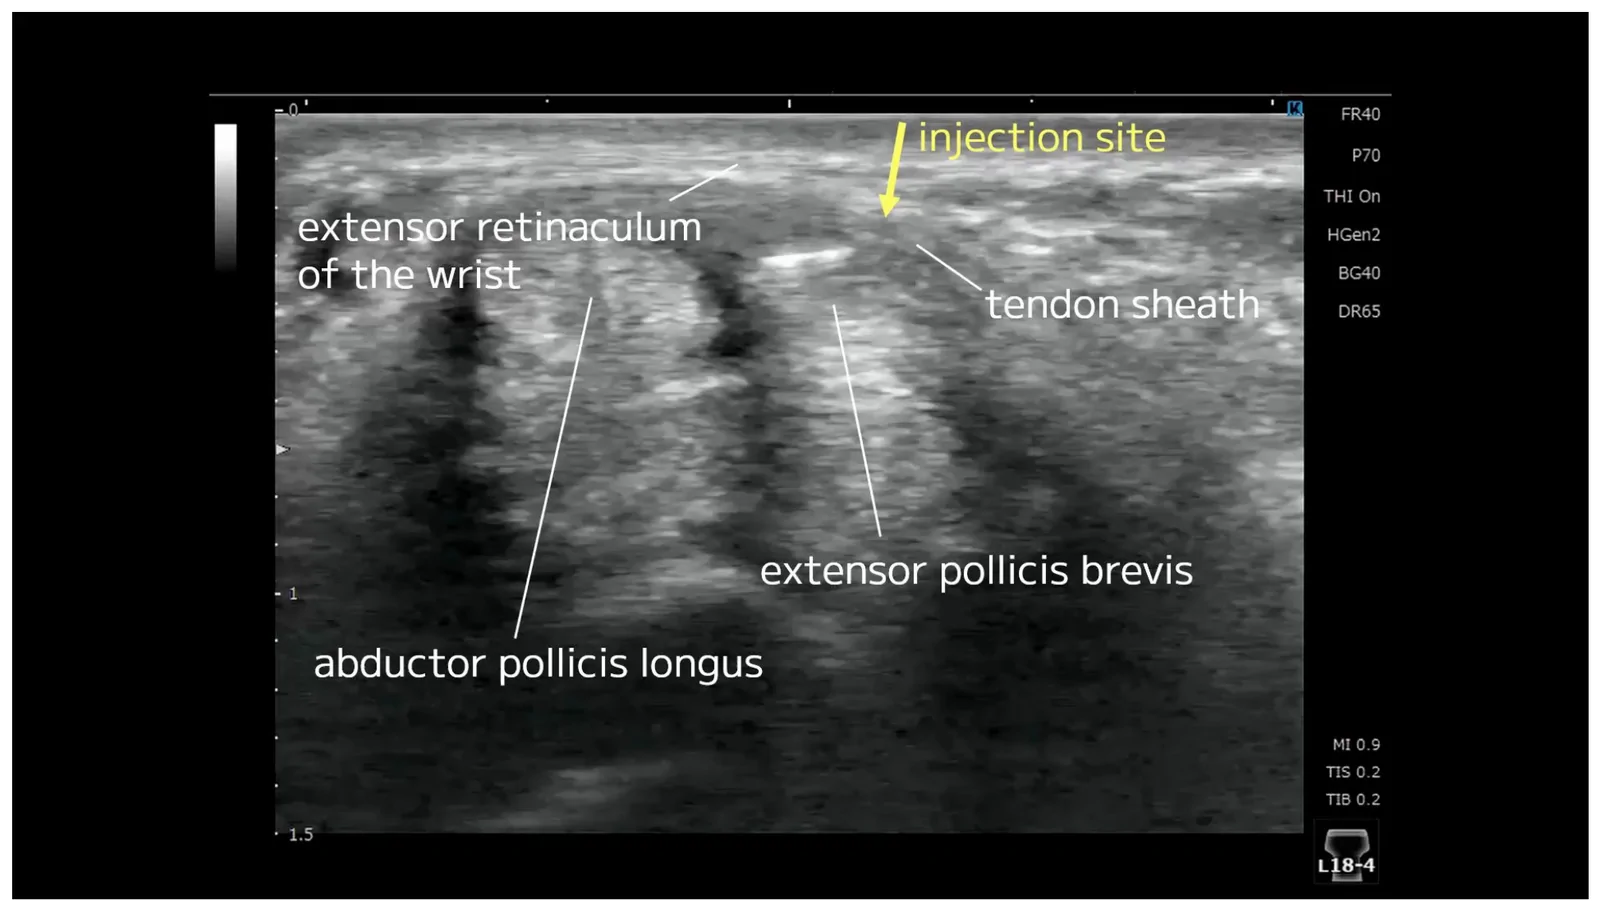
Point 1—first extensor compartment (APL/EPB): ultrasound-guided fascia hydrorelease; yellow arrow indicates the injection site.
